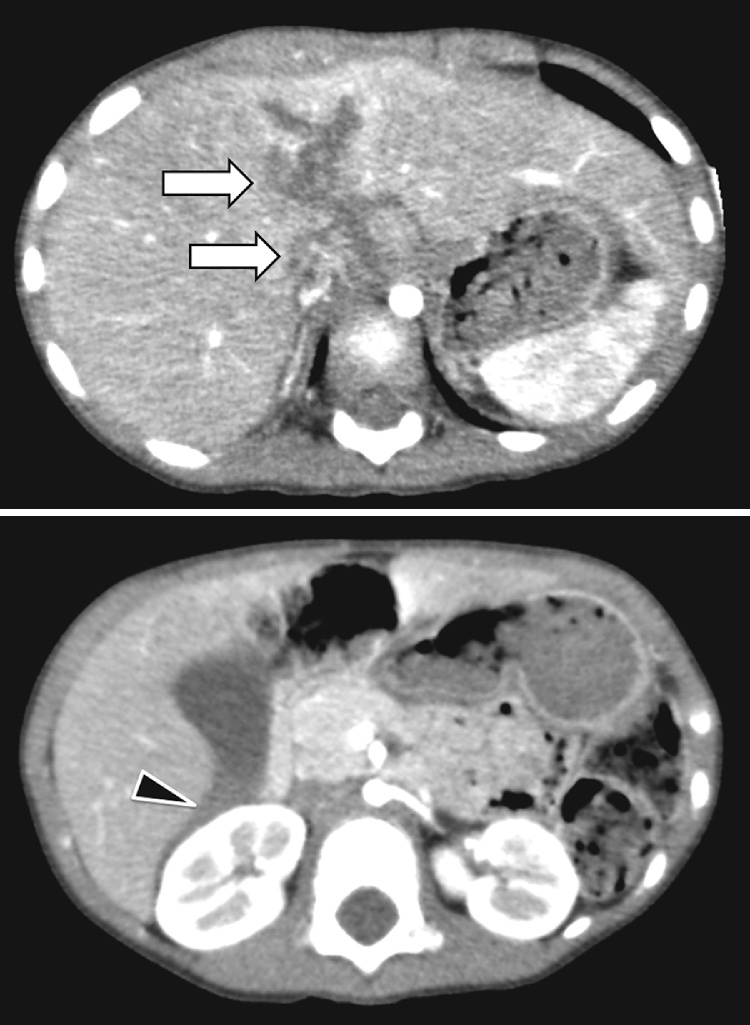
Enhanced CT one hour after admission. a) AAST Grade 4 liver injury b) Fluid collection was observed in Morison’s pouch.
